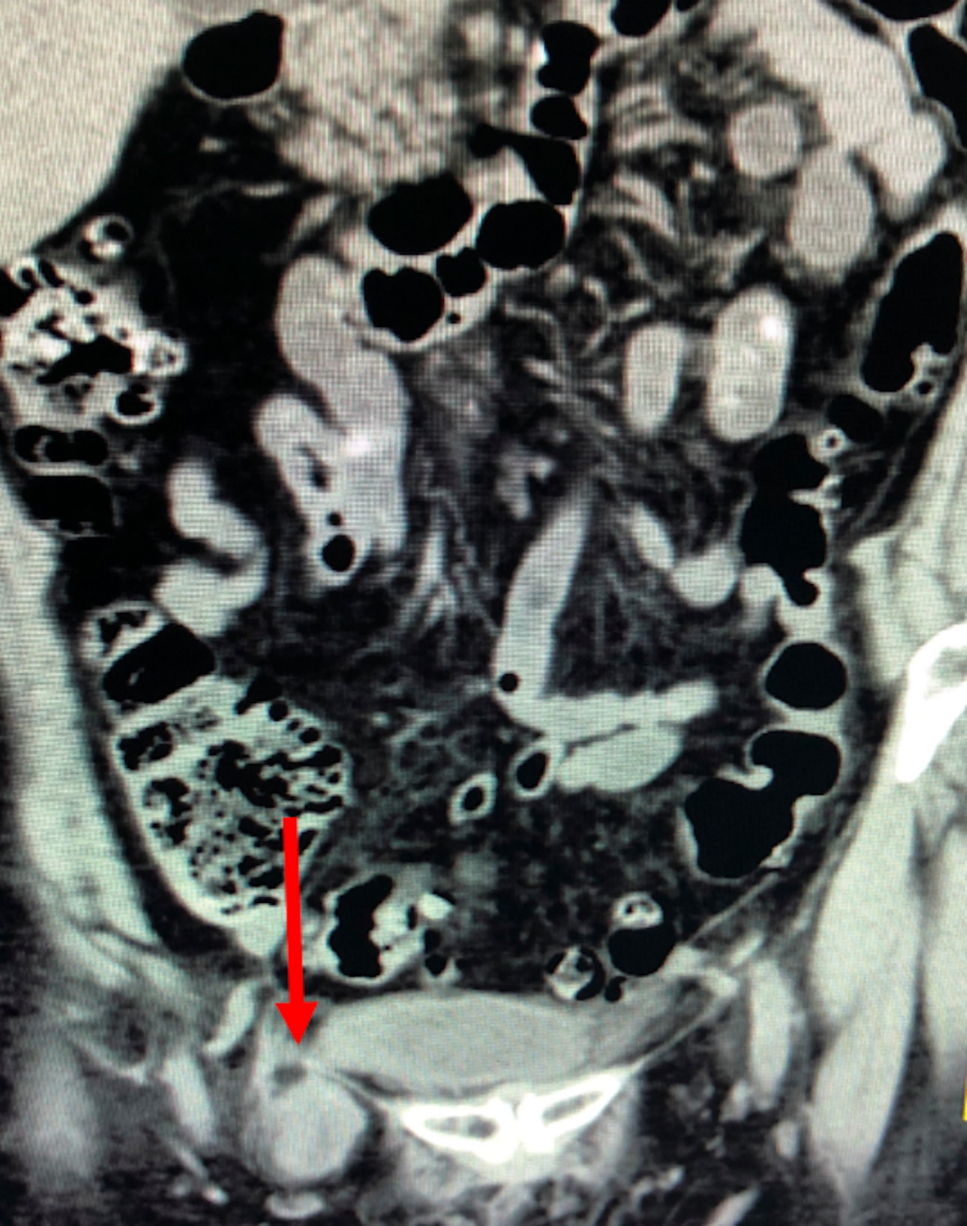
CT abdomen/pelvis coronal view: the arrow indicating herniated bladder
